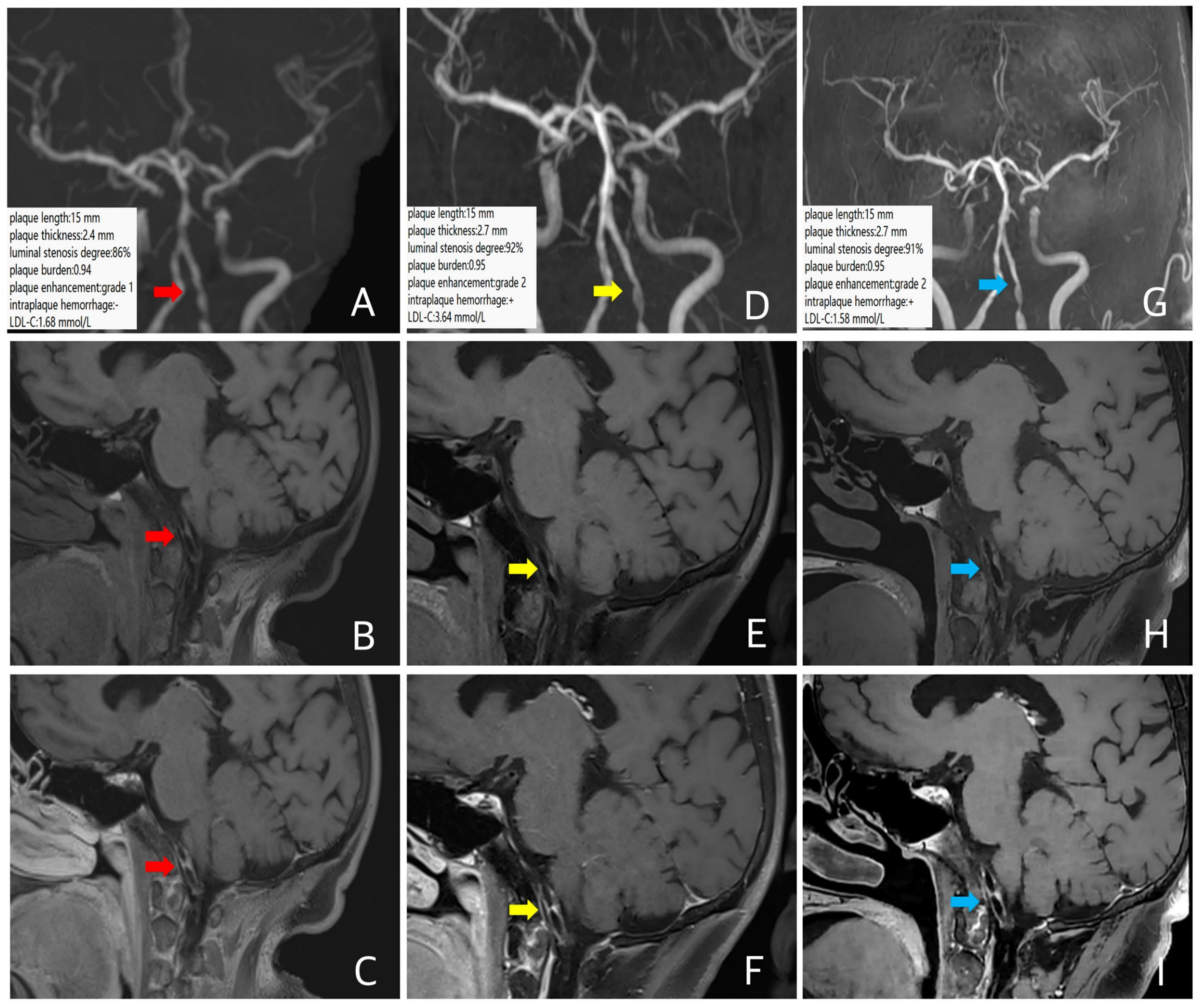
In case 2, patient at baseline (red arrow), follow-up images 11 months later (yellow arrow), and follow-up images 30 months later (blue arrow). Three-dimensional time-of-flight cerebral magnetic resonance angiography showed significant stenosis of the intracranial segment of the left vertebral artery (A,D,G). Coronal T1 plain scan weighted imaging (B,E,H) and enhanced imaging (C,F,I) in whole brain magnetic resonance imaging using different inversion angles to optimize contrast showed multiple atherosclerotic plaque formation in the left vertebral artery plaque with obvious stenosis and obvious plaque enhancement. The 11-month follow-up intracranial VW-MRI showed no significant changes in plaque length and plaque thickness compared with baseline, a slight increase in the luminal stenosis and the plaque burden, a new intraplaque hemorrhage occurred, and the plaque enhancement upgrades (D–F), indicating a progressive tendency in the plaque. Which LDL-C level is elevated. At 30 months, intracranial VW-MRI showed no significant changes in plaque compared with the second examination (G–I), in which LDL-C levels decreased.
